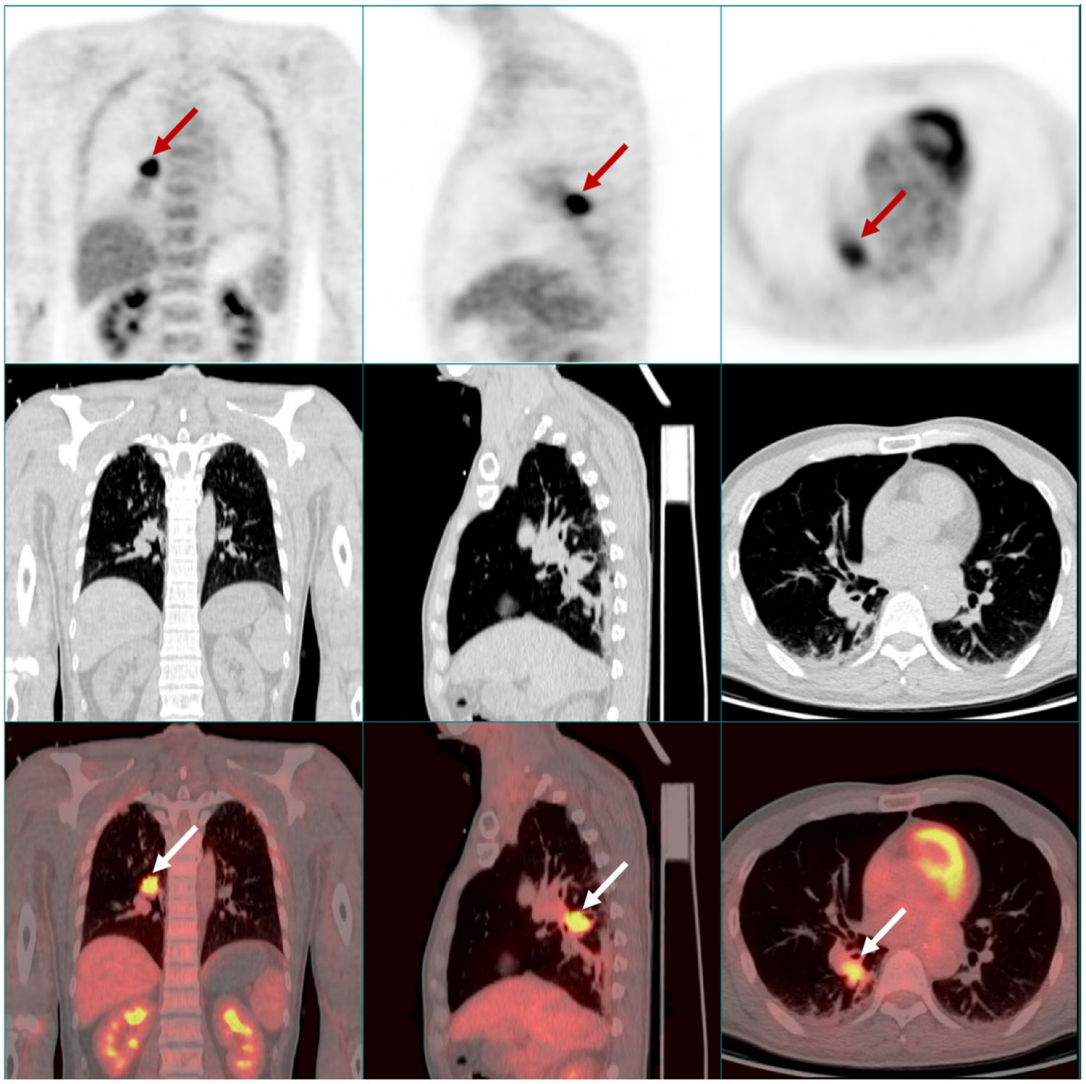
Contrast-enhanced CT and PET-CT images from a 54-year-old male patient. PET-CT showed a soft tissue mass in the inferior lobe of the right lung with abnormal metabolism (arrows), which was not evident on CT scan.
